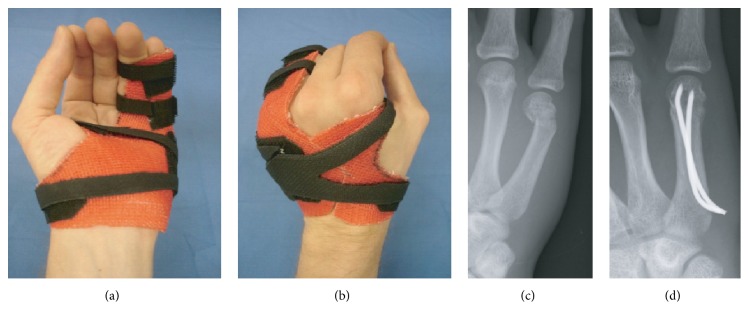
Middlehand orthoses (fingers included): (a) palmar and (b) dorsoradial view; case sample: angulated fracture of the 5th metacarpal head, (c) X-ray on admission and (d) at 6 weeks following anterograde nailing technique (2x intramedullary 2 mm k-wire) (DASH analysis of case series: *n* = 17; score-mean = 32.37 ± 2.53 SD; DASH follow-up interval (weeks): 11.68 ± 1.17 SD).
